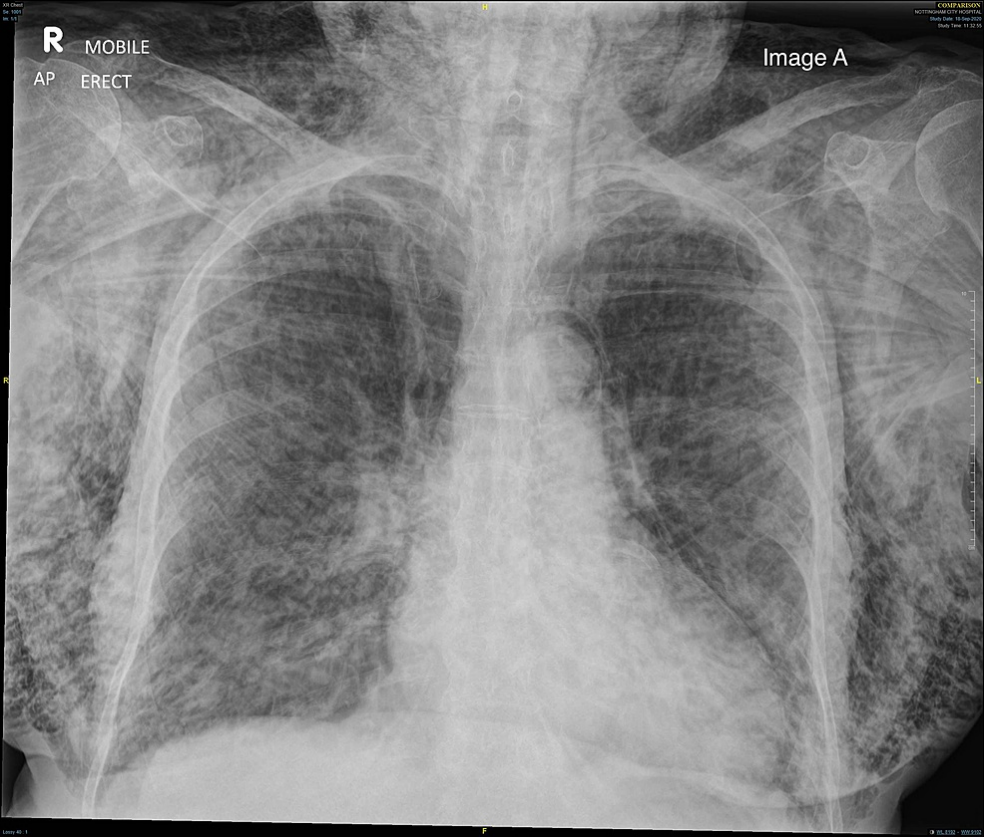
Subcutaneous emphysema chest X-ray
